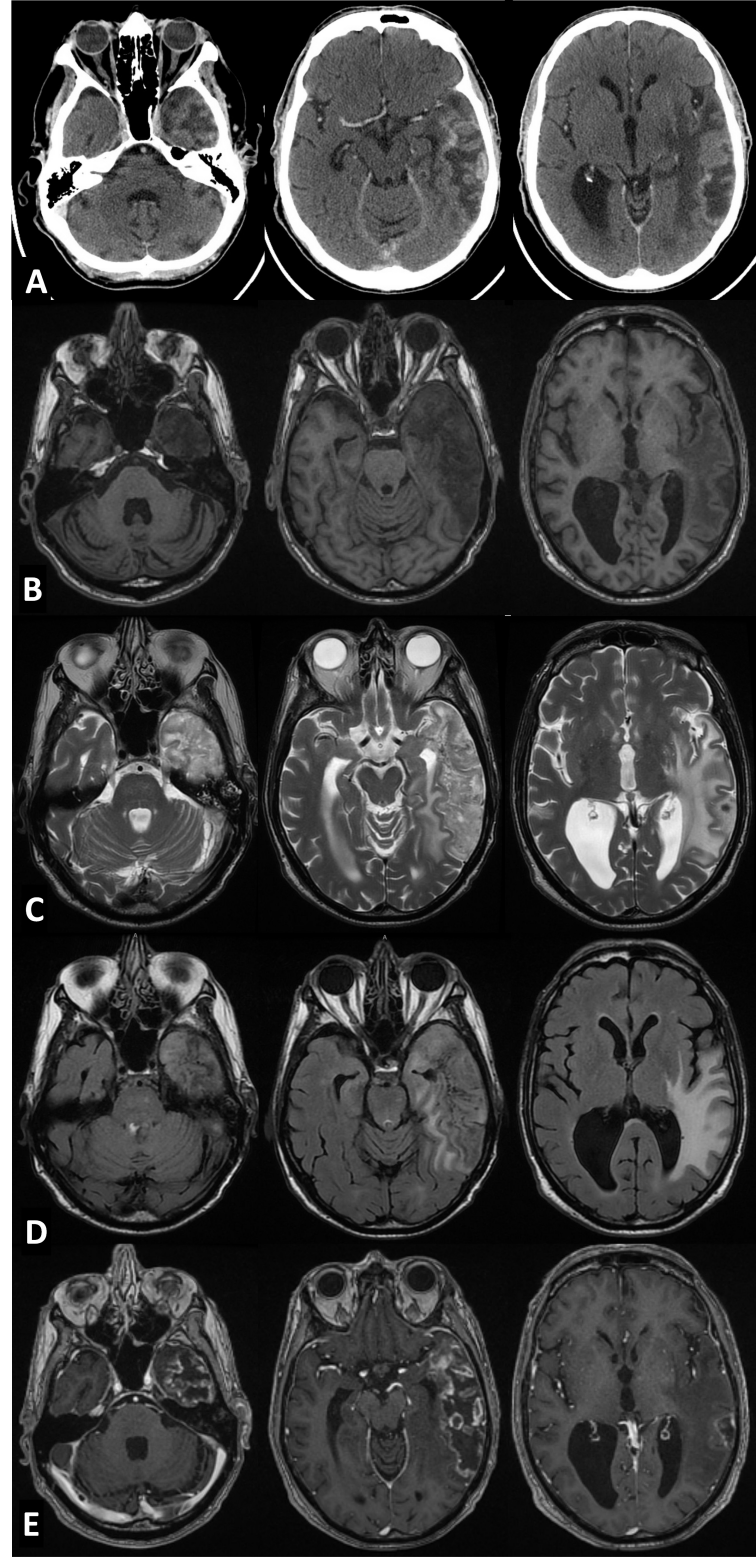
Incidental imaging findings of the patient A- Enhancing lesion with central necrosis on contrast-enhanced CT scan. B- Hypo-intense lesion with effacement of temporal horn of the lateral ventricle on left side on T1 W images. C- Hyper-intense lesion with areas of cystic degeneration T2 W images. D- Extent of vasogenic edema seen on T2-FLAIR images. E- Ring enhancement with central necrosis on post contrast images.
